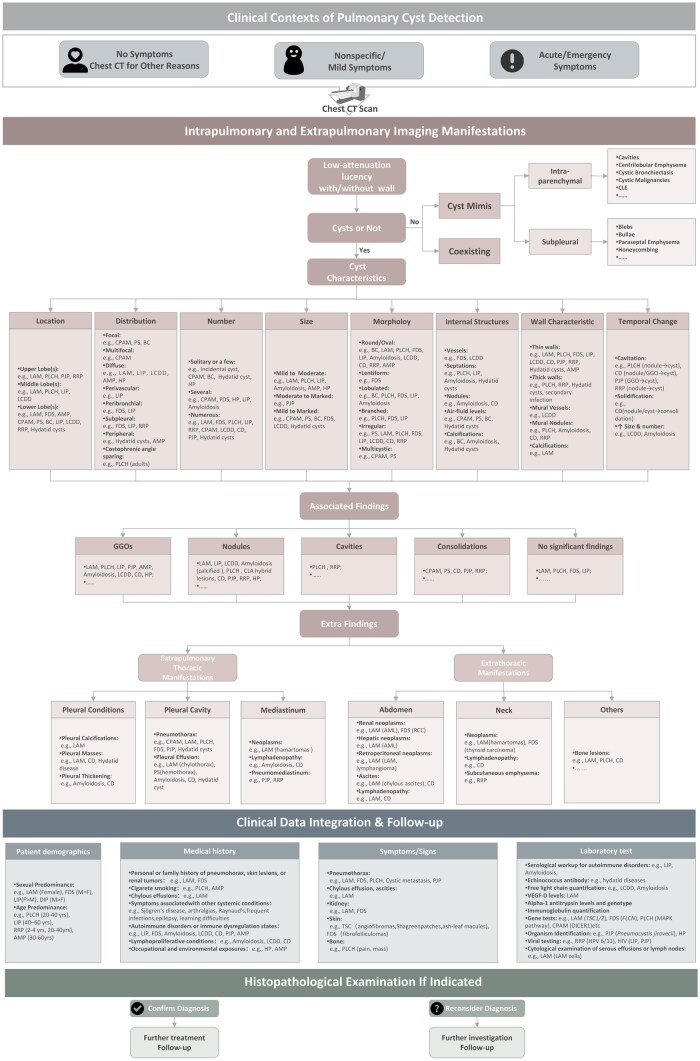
A hierarchical diagnostic approach for cystic lung diseases based on CT. Cyst size was classified as *mild* (≤20 mm), *moderate* (20-80 mm), or *marked* (>80 mm). Abbreviations: AML = angiomyolipoma; AMP = alveolar macrophage pneumonia; BC = bronchogenic cyst; BMD = bone mineral density; CD = Castleman disease; CLE = congenital lobar emphysema; CLM = congenital lung malformations; CPAM = congenital pulmonary airway malformation; FDS = folliculin deficiency syndrome; GGO = ground-glass opacity; HP = hypersensitivity pneumonitis; LAM = lymphangioleiomyomatosis; LCDD = light chain deposition disease; LIP = lymphoid interstitial pneumonia; PJP = *Pneumocystis jirovecii* pneumonia; PLCH = pulmonary Langerhans cell histiocytosis; PS = pulmonary sequestration; RRP = recurrent respiratory papillomatosis; TSC = tuberous sclerosis complex; VEGF = vascular endothelial growth factor.
